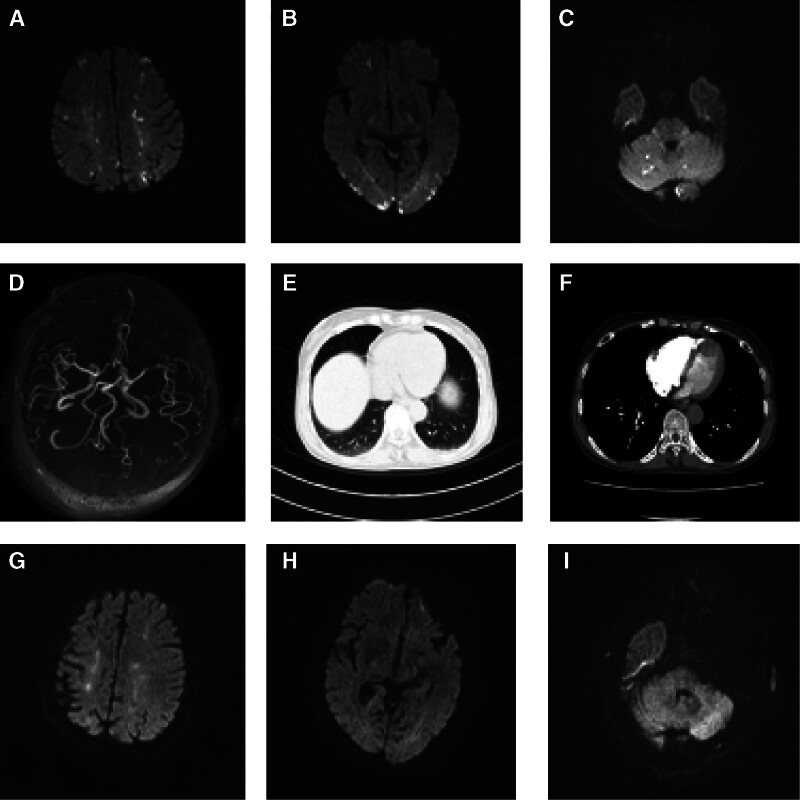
DWI at onset (A–C): Diffuse multiple spot-like hypersignals were observed in bilateral frontal, parietal, temporal, occipital, lobes, center semicovale, and bilateral cerebellum, thereby presenting a typical “Three Territory Sign” change. MRA (D): cerebral artery stiffness and no obvious stenosis. Lung CT (E): Patchy exudate shadows were observed under the pleura in the lower lobes of both lungs with unclear boundaries. Lung CTA (F): Multiple filling defective shadows were observed in some arterial branches of the lower lobe of both lungs. DWI at discharge (G–I): the lesions with limited diffusion were significantly less than before. CTA = computed tomography angiography, DWI = diffusion weighted imaging, MRA = magnetic resonance angiography.
